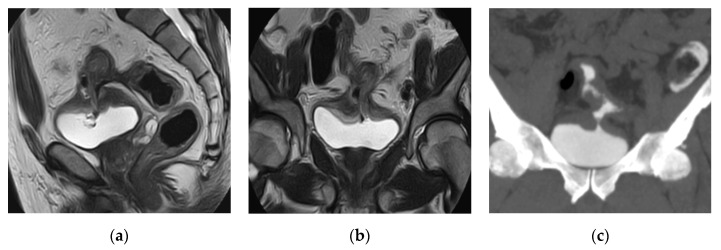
Colovesical fistula. (a) Sagittal and (b) coronal T2 weighted images show marked irregular bladder wall thickening at the bladder dome with related inflammatory soft tissue closely related to the adjacent thick-walled sigmoid colon. Intravesical signal void air locules also could be noted. (c) Coronal reformatted CT cystography confirms the presence of colovesical fistula.
